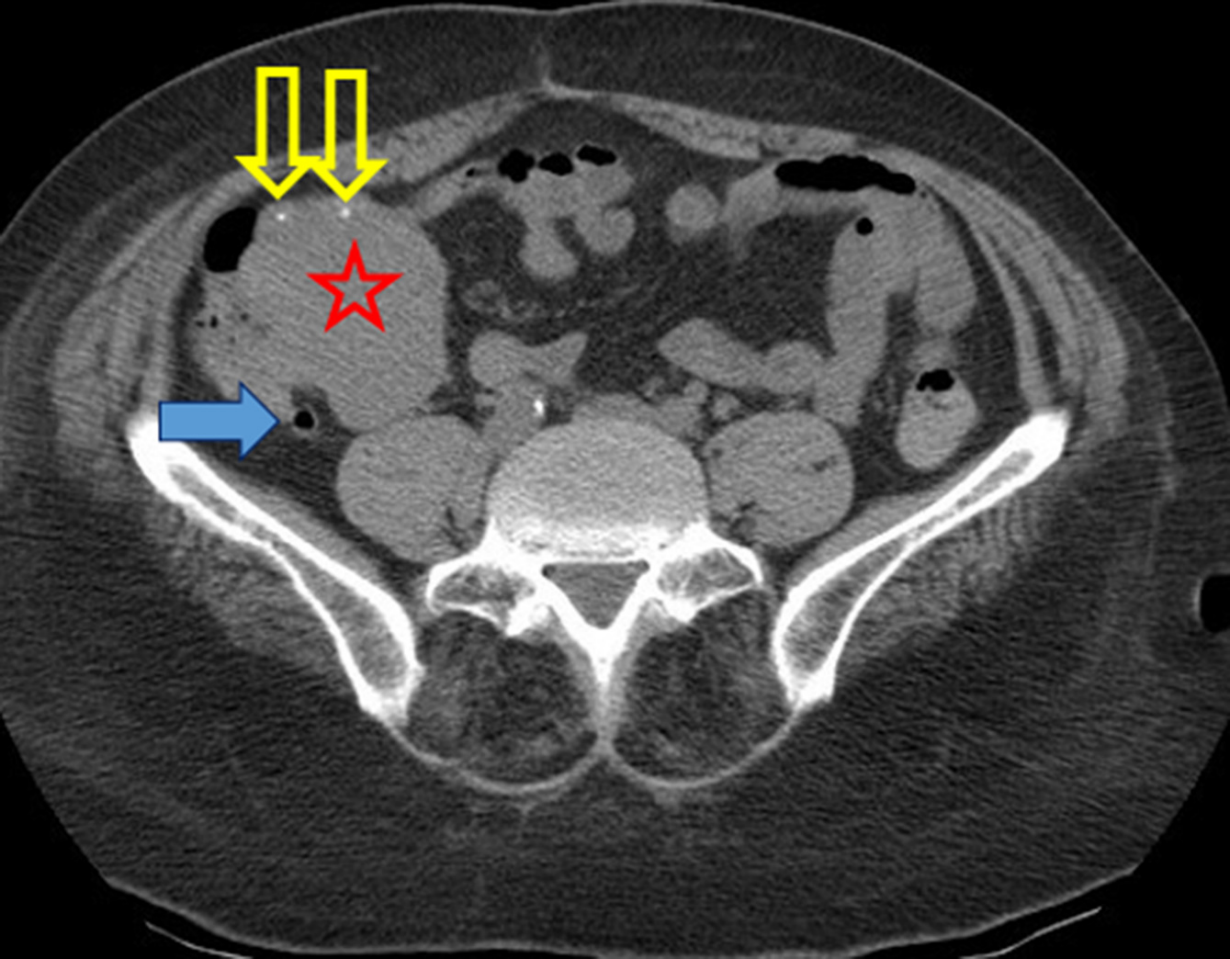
Axial non-enhanced CT scan. Large, isodense lobulated mass (red star) at the ileocecal junction with some small calcifications (open yellow arrows). Notice the normal air-filled appendix (blue arrow).
